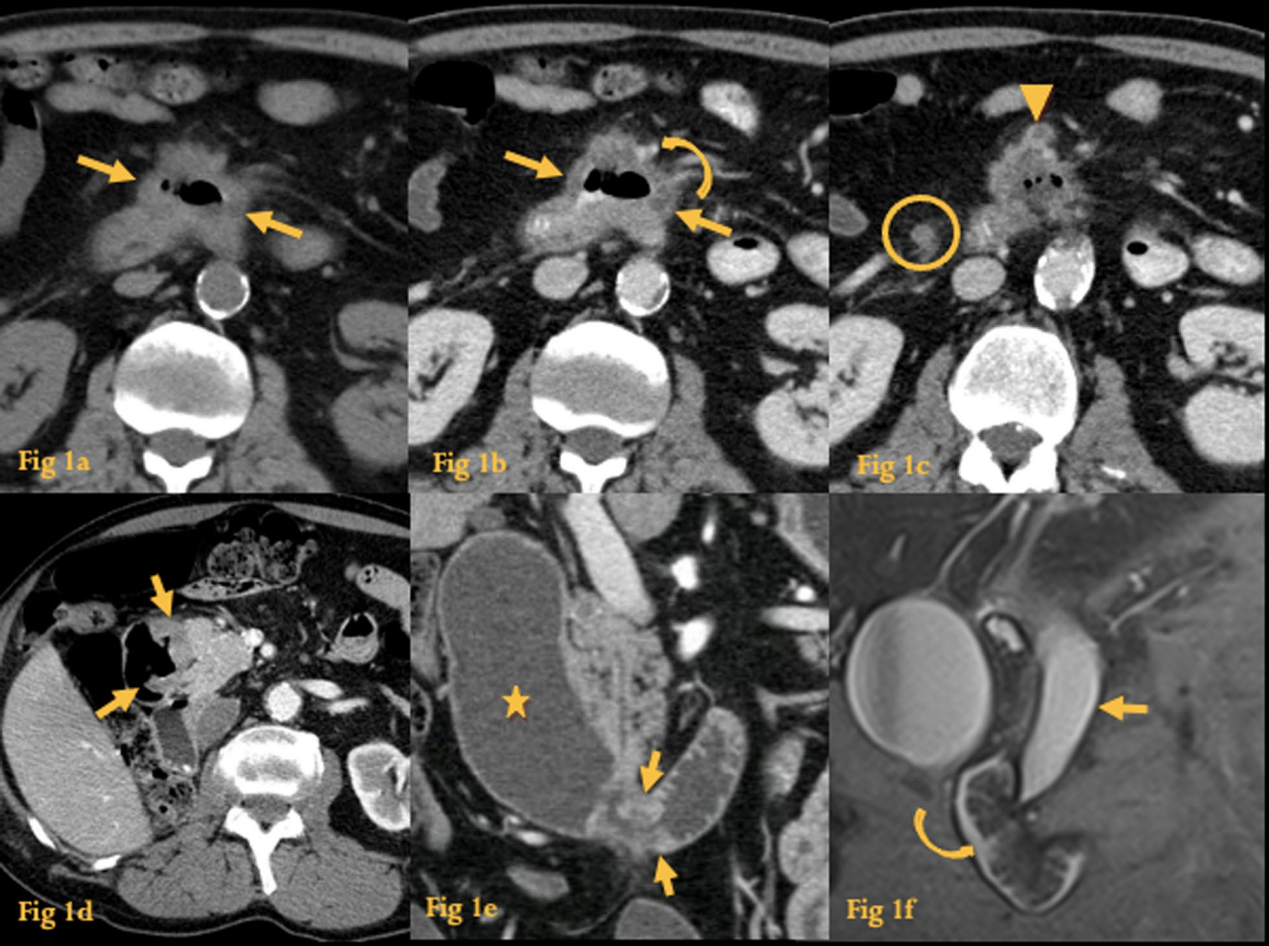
Adenocarcinoma. Abdominal CT performed before (a) and after (b, c) iv contrast administration shows irregular circumferential asymmetric thickening of D3, causing deformity of the lumen (arrows). There is encasement of the superior mesenteric artery (curved arrow) and tumor thrombosis of the superior mesenteric vein (arrowhead), rendering the lesion surgically unresectable. There is also periduodenal fat stranding and adenopathy (circle). Abdominal CT (d) of another case of duodenal carcinoma with an ulcerated mass at D2 (arrows). Abdominal CT of yet another case of duodenal carcinoma (e); there is circumferential duodenal thickening (arrows) which caused luminal stenosis with proximal dilatation (asterisk). MRCP of another patient (f) showing a polypoid duodenal lesion (curved arrow) that caused CBD dilatation (arrow), which proved to be a duodenal adenocarcinoma
